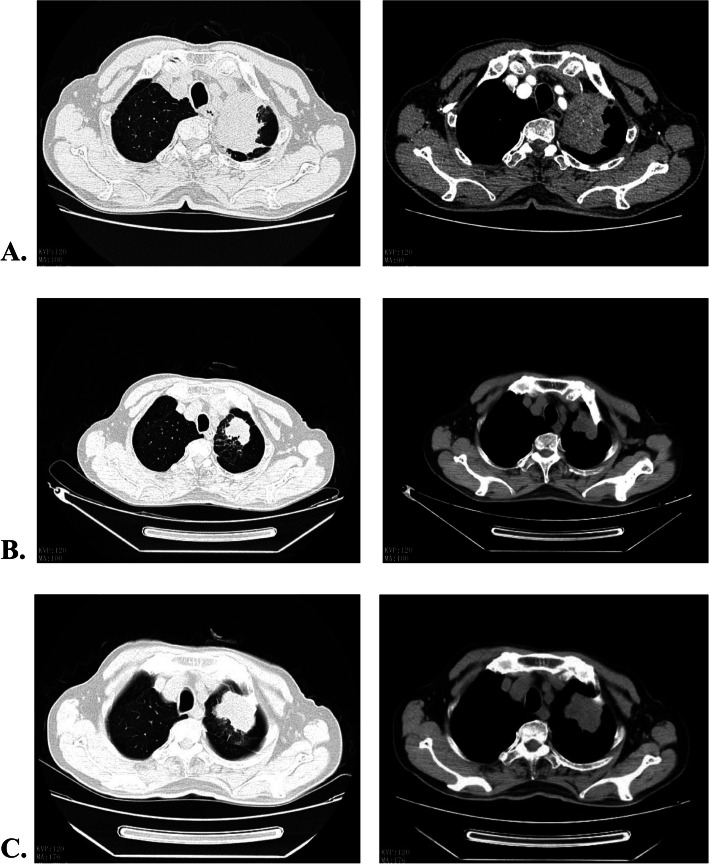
Computed tomography scans of the patient showing the tumor responses to icotinib target therapy. a Scans at the baseline when diagnosed with primay myoepithelial carcinoma of the lung on Jul. 17, 2019 (tumor diameter of 6.2 cm × 4.7 cm). b Scans after icotinib regimen, with the tumor diameter of 4.2 cm × 3.5 cm and disease evaluated as PR on Sep. 07, 2019. c Scans during disease progression with tumor diameter of 4.8 cm × 4.7 cm on Oct. 07, 2019
